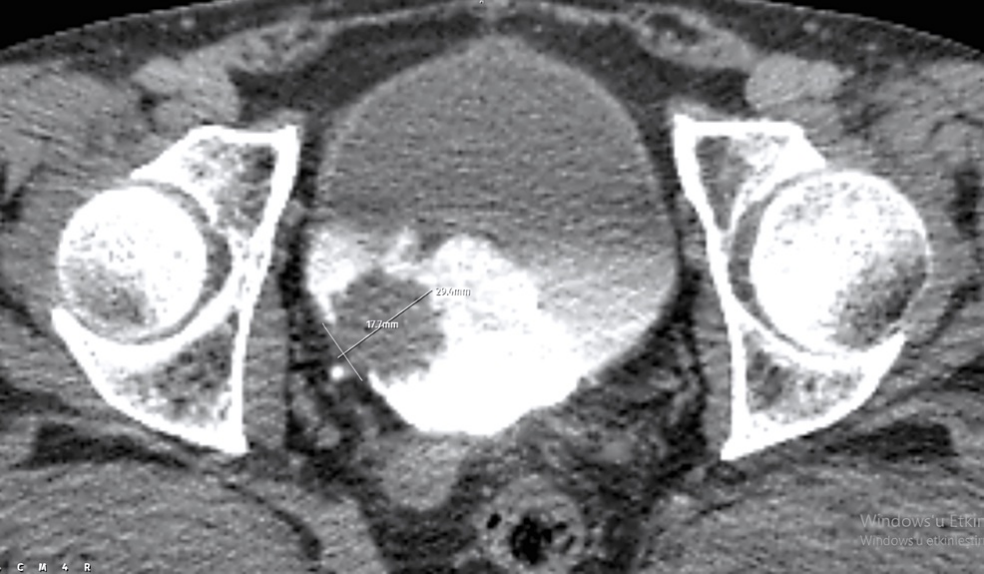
Axial CT section of the bladder tumor in a 56-year-old male patient.
